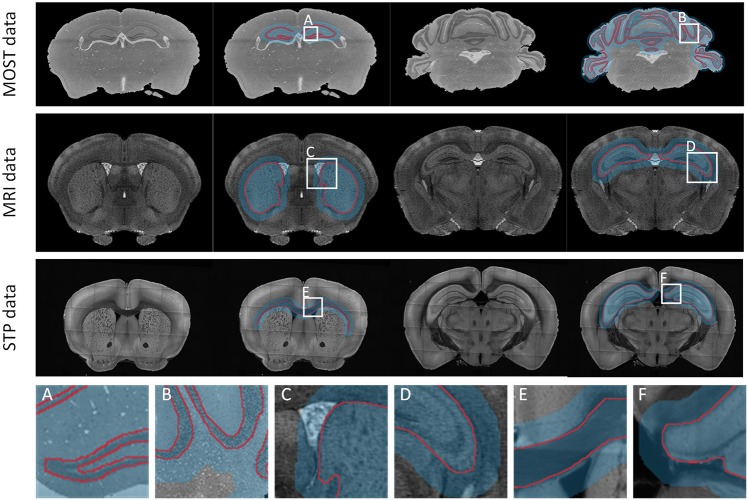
Applicability of DeepBrainSeg for other types of datasets. The first row shows the segmentation effects of HIP-pg and CBX-gl for MOST data. From left to right: the coronal image, the superposition of image, the localization masks, and the segmented lines for HIP-pg and CBX-gl. The second and third rows show CP and HIP for the MRI data, CC, and HIP for STP data, respectively. (A–F) Enlarged views of the areas in white boxes in the first three rows.
